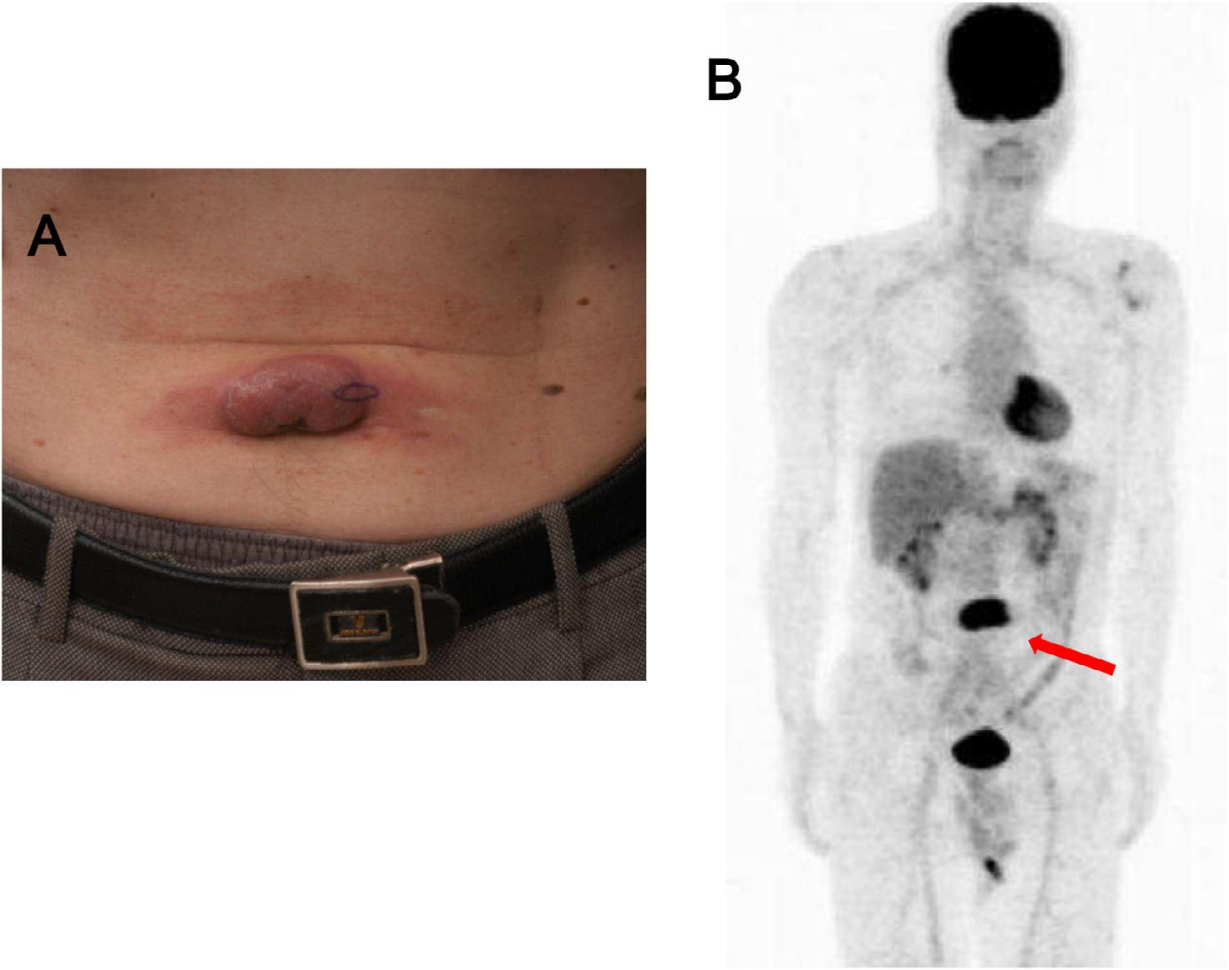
(A) Clinical appearance of patient No. 42. (B) 18F‐fluorodeoxyglucose (FDG) positron emission tomography (PET) imaging shows FDG uptake in the lesion (arrow).
